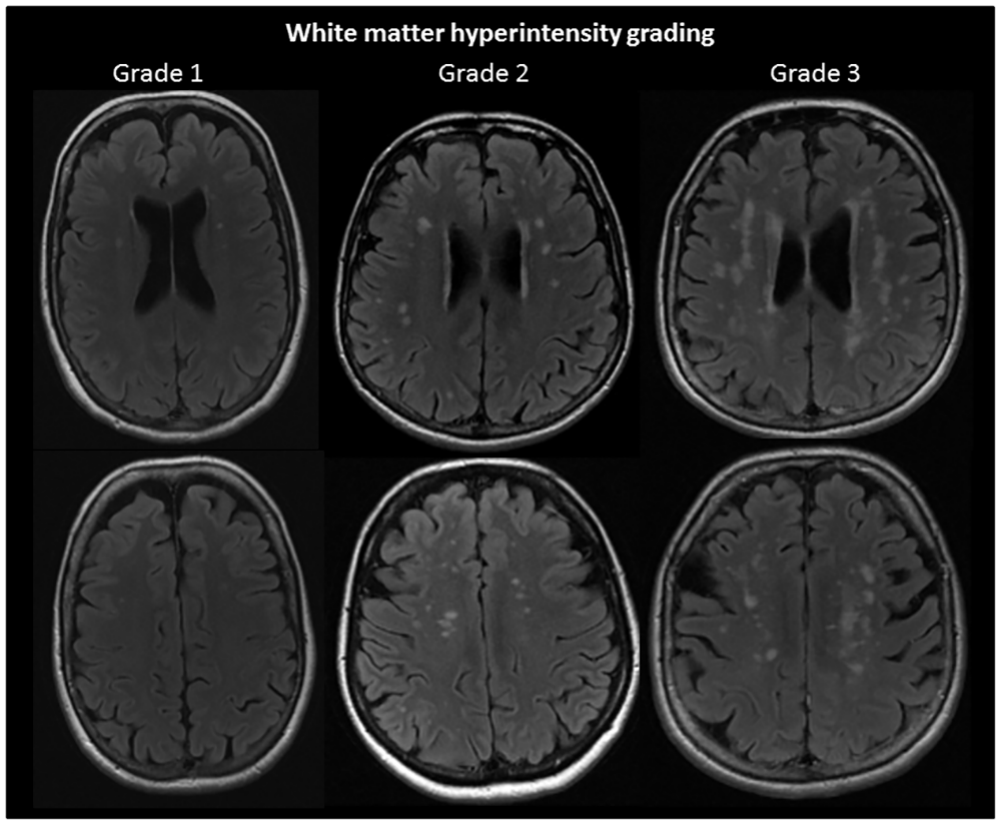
Typical examples of Fazekas grade 1, 2 and 3 white matter hyperintensities (WMH). The examples are from the FLAIR scans of three HUNT MRI participants.
